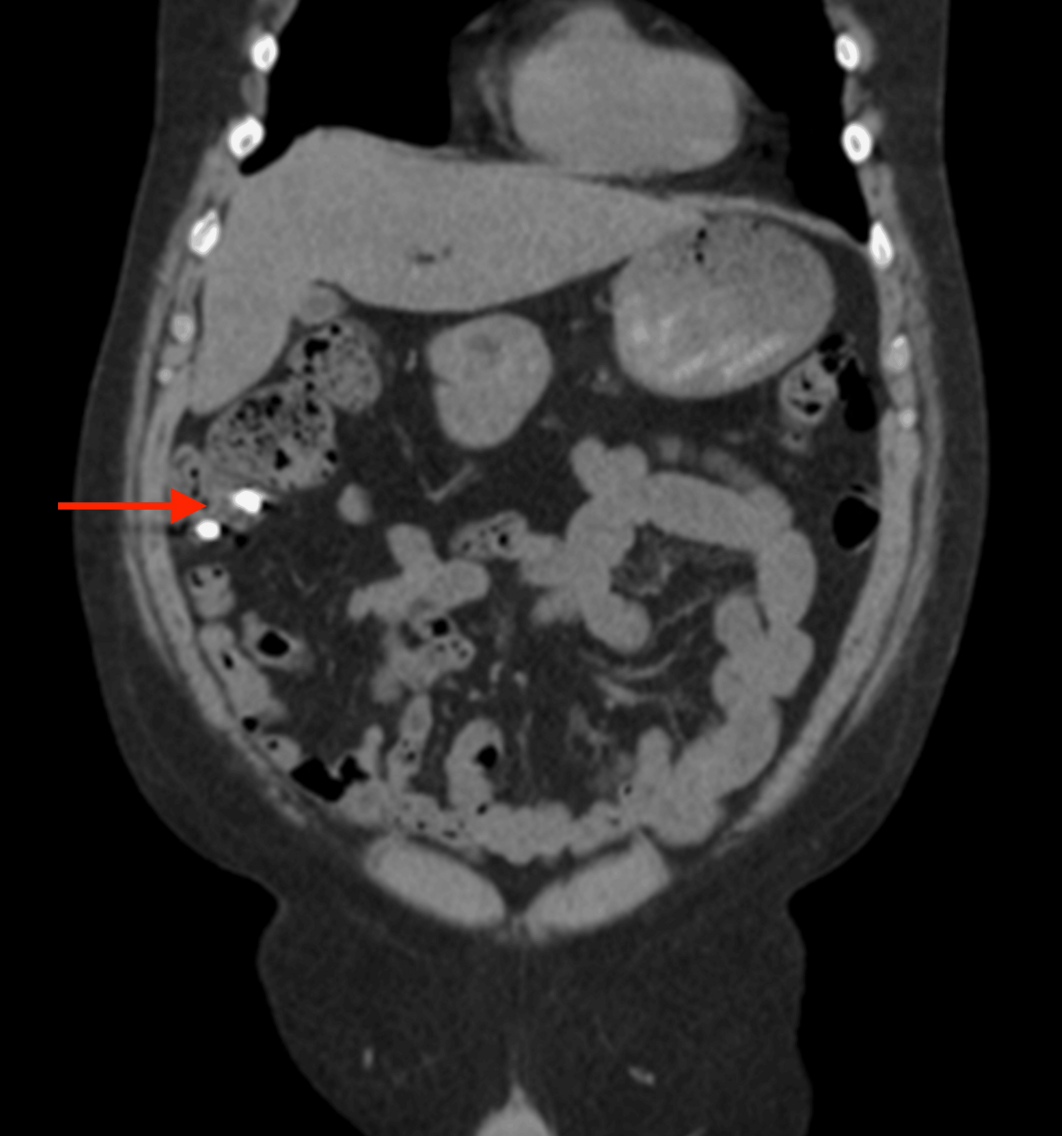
Computed tomography of the abdomen without contrast agents showing multiple sub-centimeter high-density foreign bodies in close approximation with each other within the cecum and ileum (red arrow). No evidence of bowel obstructions or bowel rupture on CT of the abdomen.
